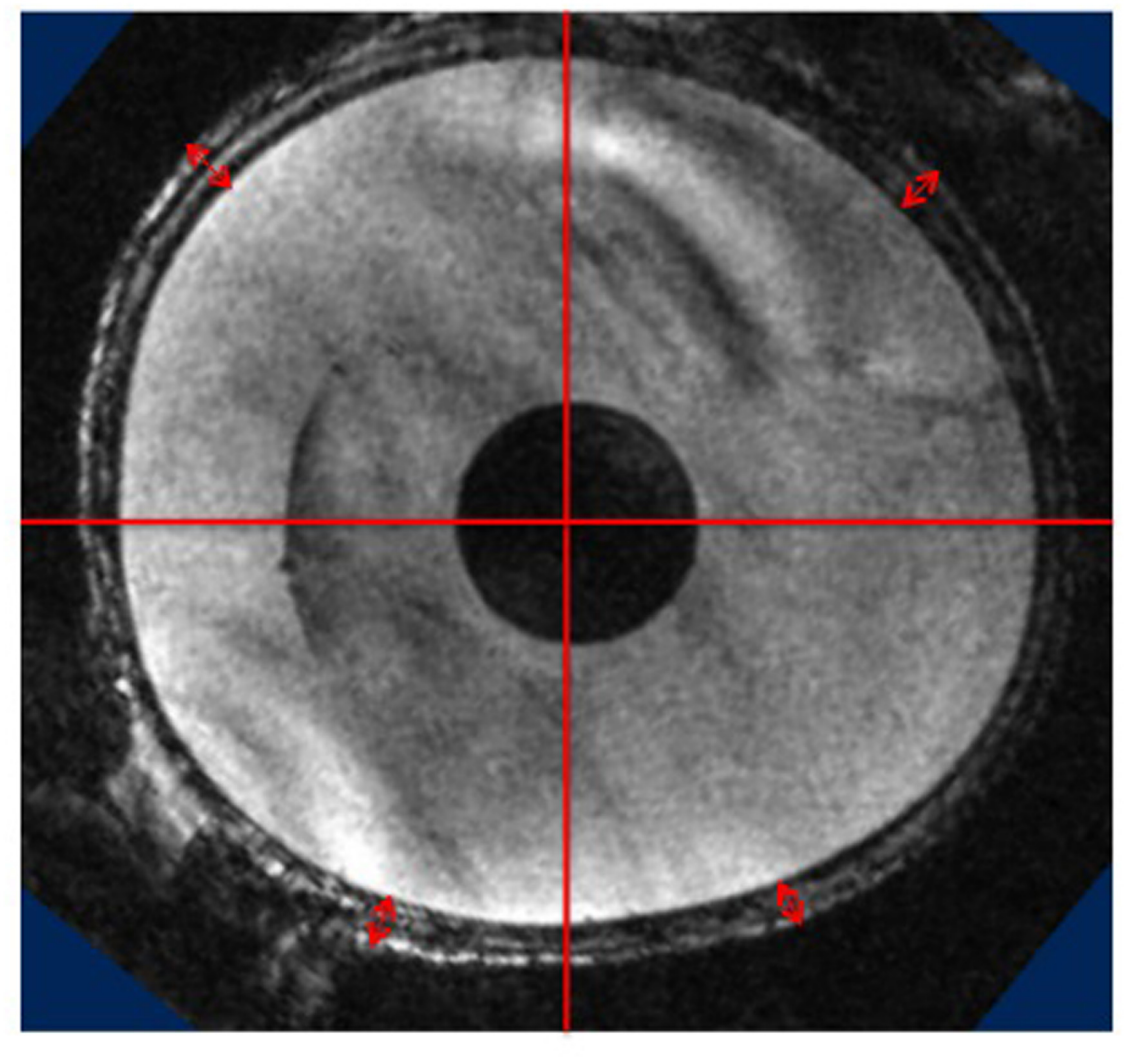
Example picture of MR image in axial plane utilized for thickness measurements. Measurements were performed in each quadrant from the shell of the implant to the outer side of the skin (red arrows). Scale was set with means of the inner circle that had the standardized diameter of 0.8 centimeter.
